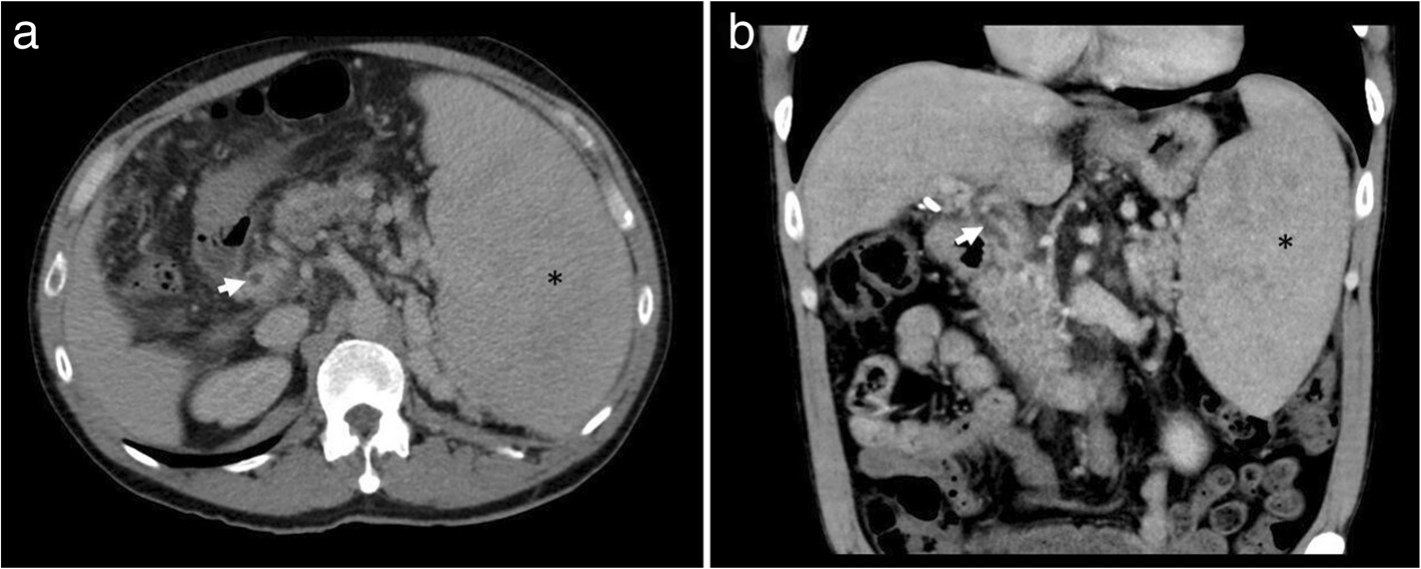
Axial (a) and coronal (b) CT demonstrating a filling defect within the portal vein (white arrows). Note the presence of splenomegaly in the patient (asterisk) and multiple splenic varices
